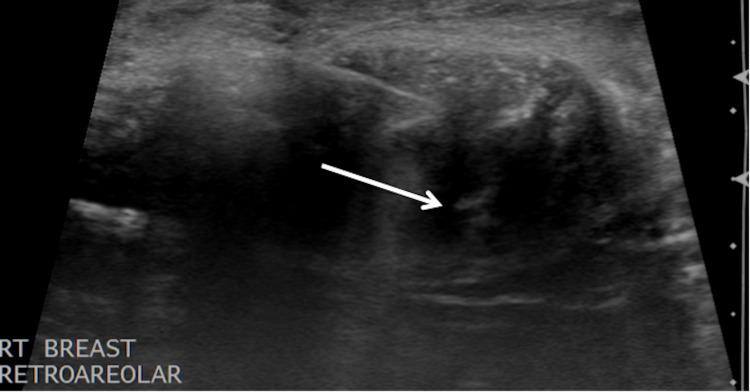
Core needle biopsy. Ultrasound-guided core needle biopsy of the right retroareolar mass. White arrowhead points at the biopsy needle.
